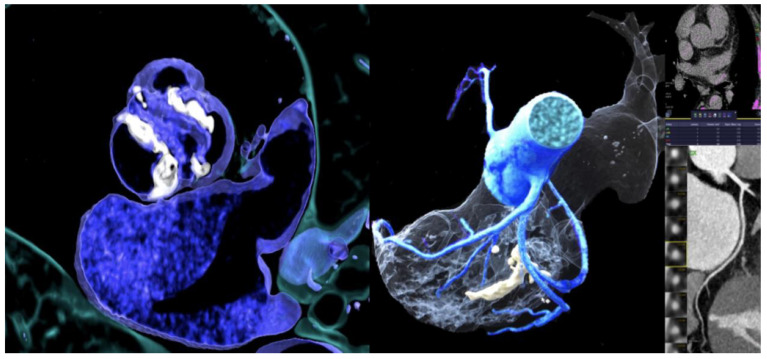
72 years-old-male with severe bicuspid AS (Type I, Raphe L/R) (left), zero CACS (right, upper) and no coronary stenosis by CTA (right).
